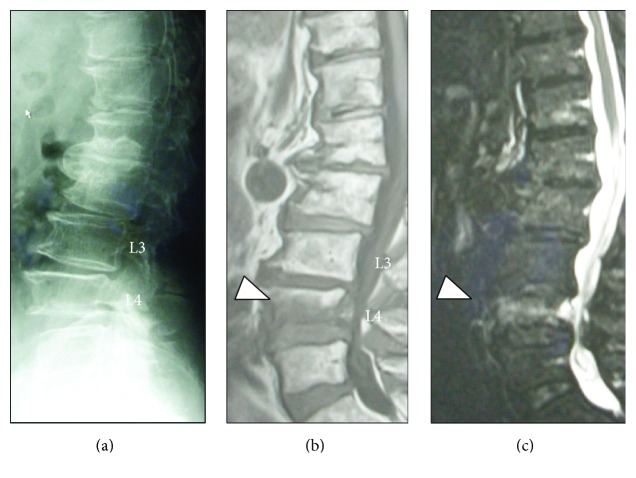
A 79-year-old woman with preosteoporosis (*T*-score = −2.0 SD) and a history of strain. Plain lumbar X-ray showed spondylosis with no apparent fracture (a). MRI showed a low intensity lesion in the T1-weighted image (b) with a high intensity change in the T2-STIR image (c) indicating an occult fracture at the L4 vertebral body (arrowheads).
